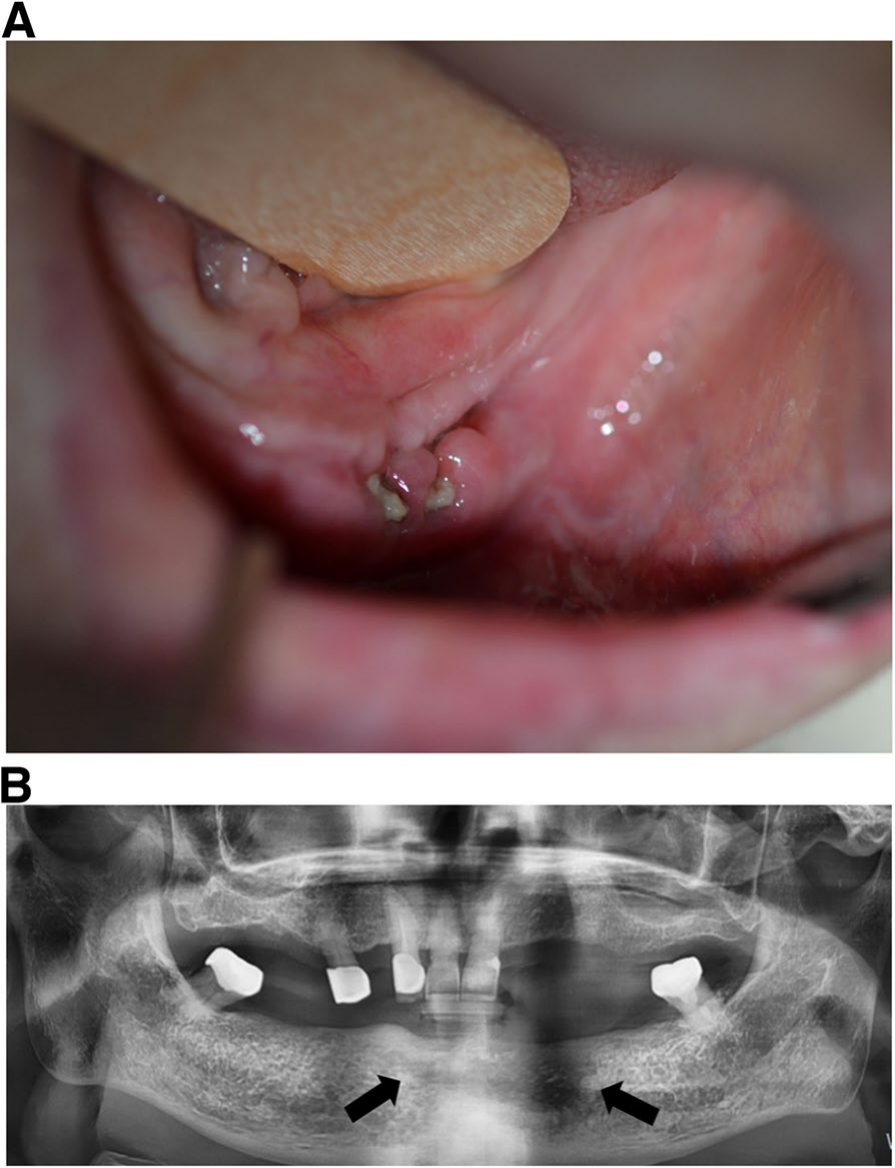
Postoperative 5 months. A Mild gingival swelling and bone exposure in the left posterior mandibular region. B Panoramic radiograph. Radiolucent periapical lesion in the left posterior mandibular region
